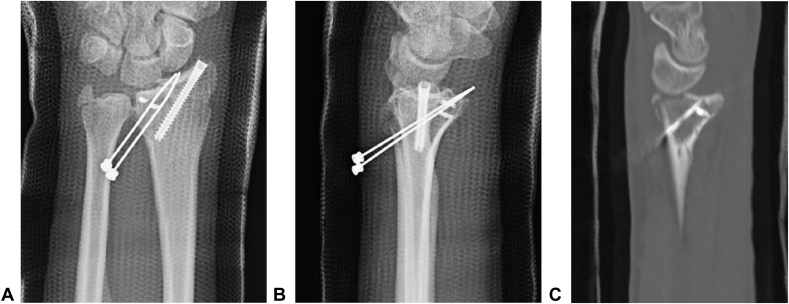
Recurrence of the dorsal radiocarpal joint dislocation. A Anteroposterior radiograph. B Lateral radiographs. C Computed tomography sagittal reformation.
